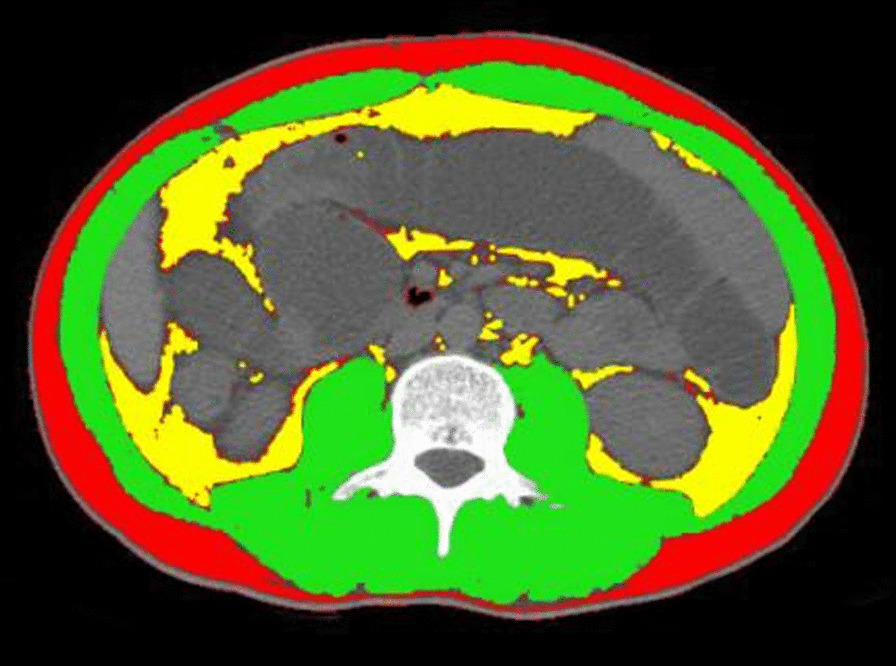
Evaluation of the body composition using a third lumbar computed tomography scan slice. Red: subcutaneous adipose tissue (SAT). Green: skeletal muscle (SM). Yellow: visceral adipose tissue (VAT)
